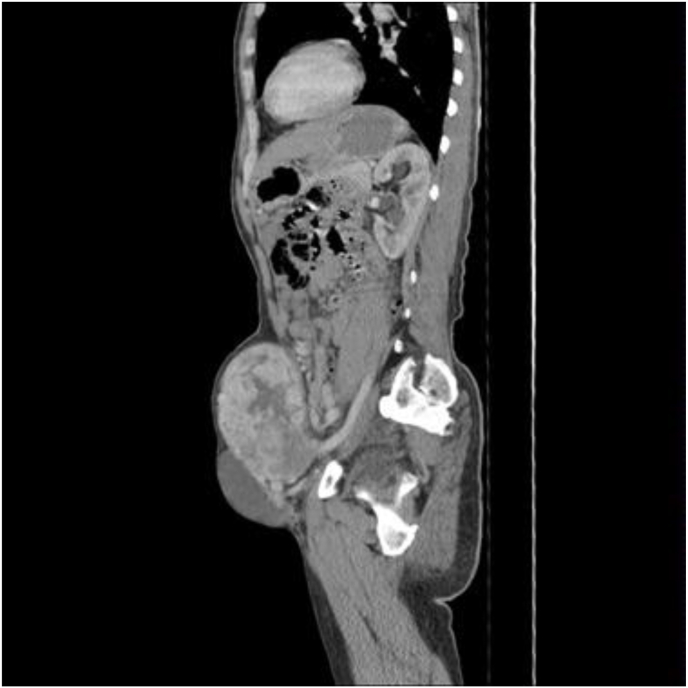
Sagittal view of a computed tomography scan showing a hypodense, heterogenous, extraperitoneal mass at the left iliac fossa, adjacent to the anterior abdomen.
